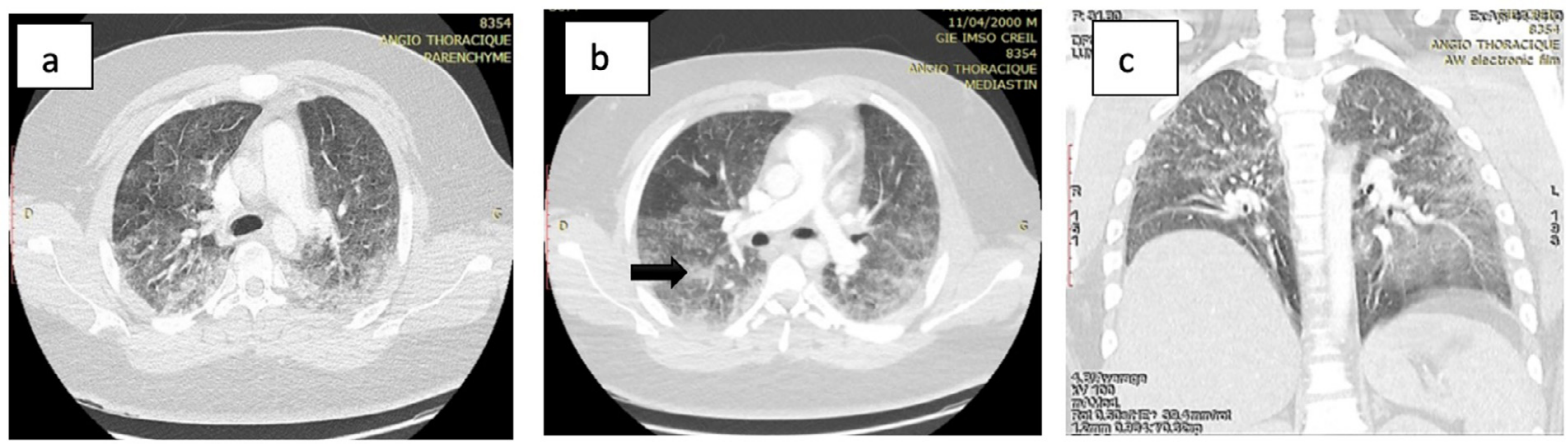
Chest CT-scan on D3. (A) Ground glass opacities and thin, bilateral reticular opacities. (B) Bilateral pulmonary condensation, posterior predominance, presence of spared healthy zones (black arrow). (C) Bilateral ground glass and thin reticular opacities affecting all the lobes.
